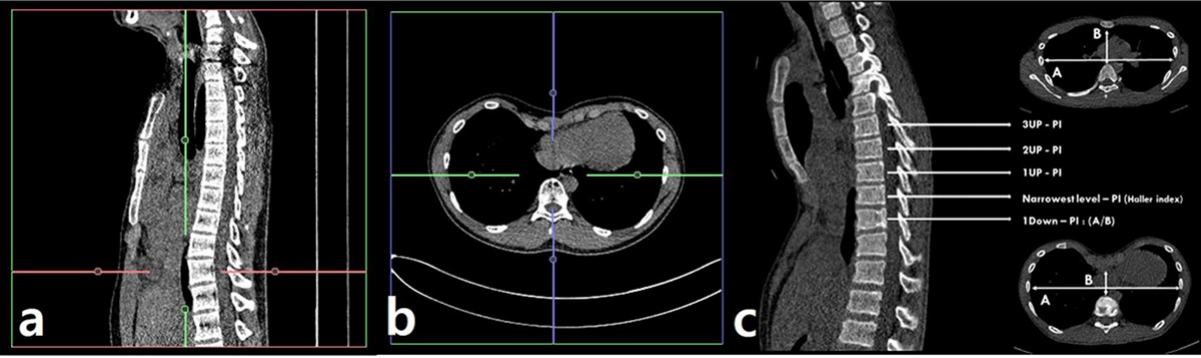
CT measurement of the vertebral-specific pectus index.
